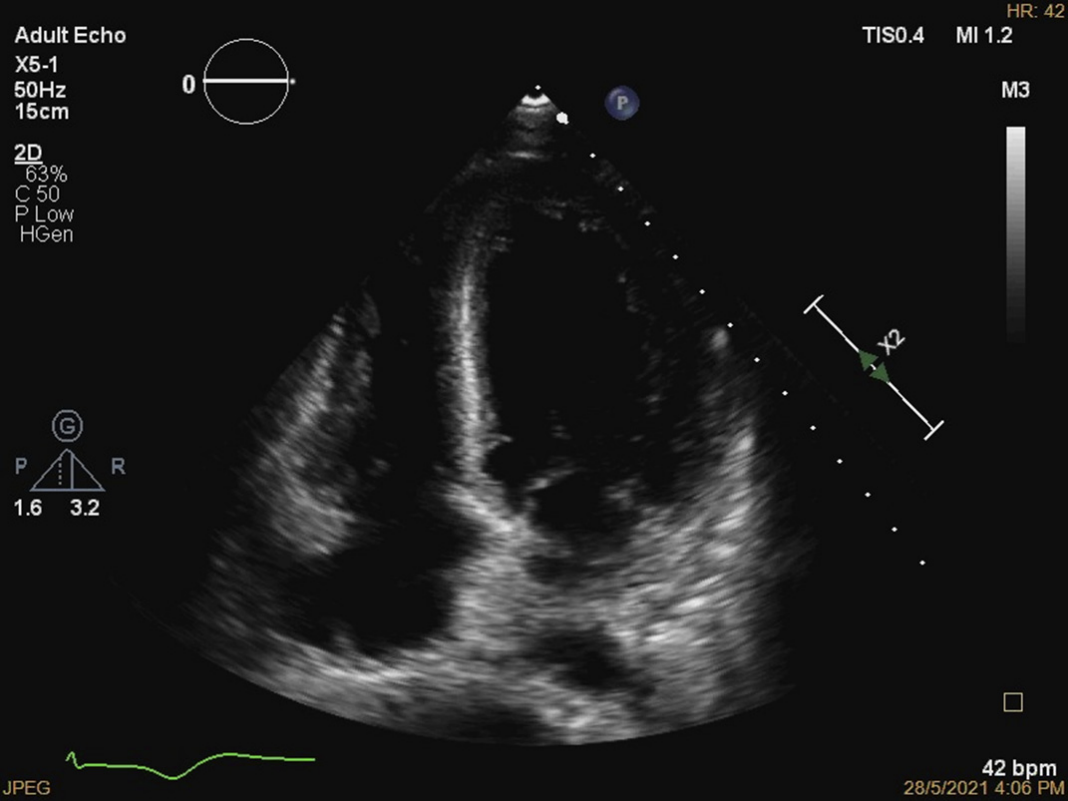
Still Image From Echocardiography Apical 4-chamber view after 3 weeks of antituberculosis treatment showing resolution of the left ventricular apical clot
